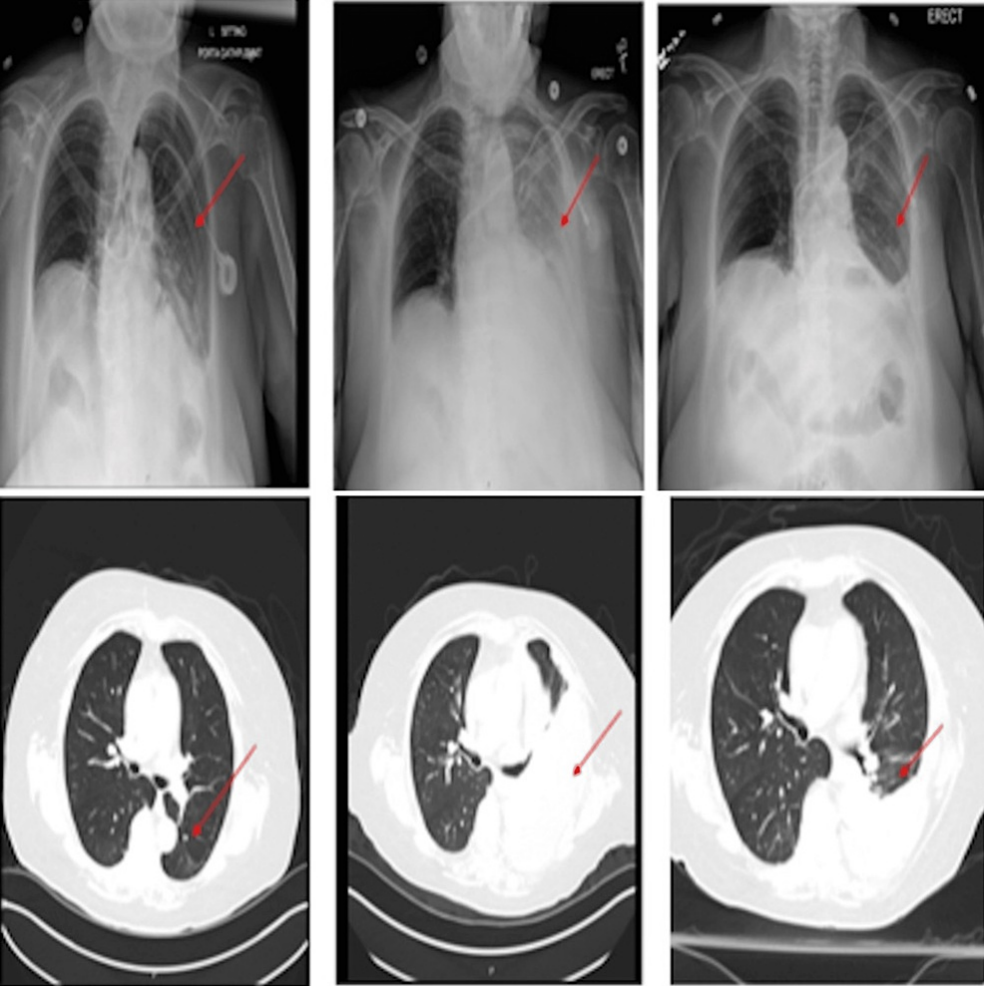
Serial chest X-ray and chest computer tomography obtained over the hospital course from left to right - Image from the day of admission (on left) showed no effusion, next image from the time patient was diagnosed with Candida empyema demonstrated the new onset of large left pleural effusion and the last images on right from post-treatment demonstrated interval resolution of empyema
